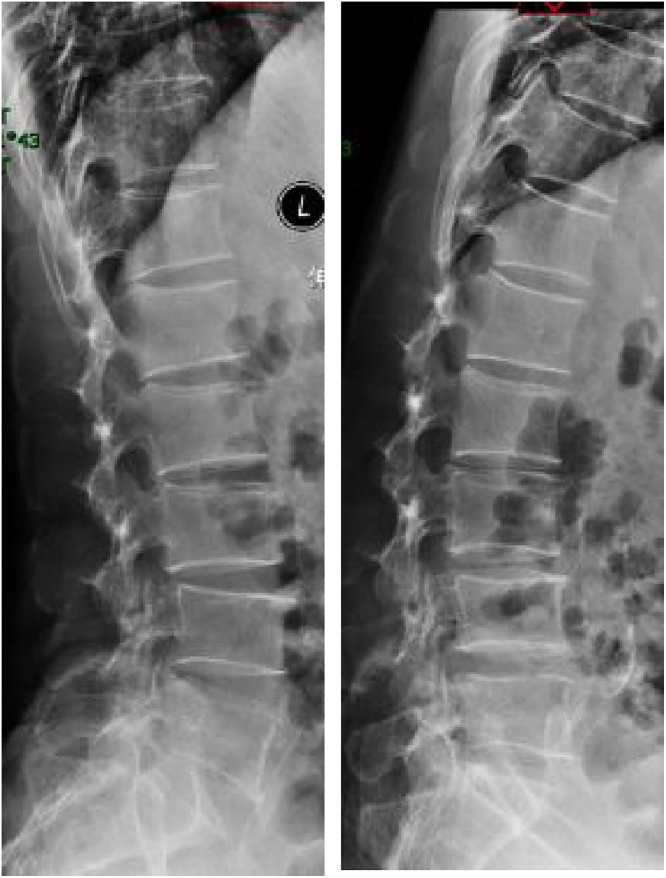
Extension and flexion X-ray showed spinal column unstable was not observed.
